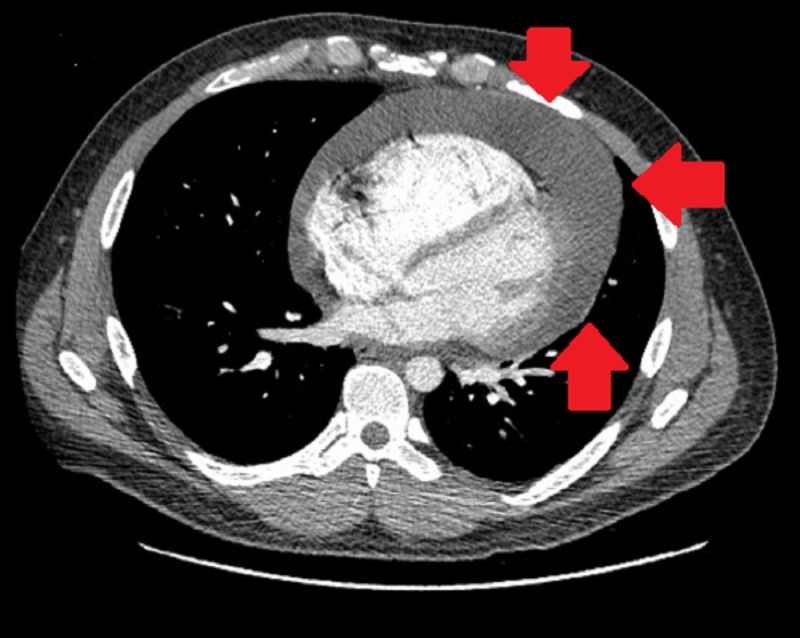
Computed tomography of the chest showing circumferential pericardial effusion and no significant pleural effusion
